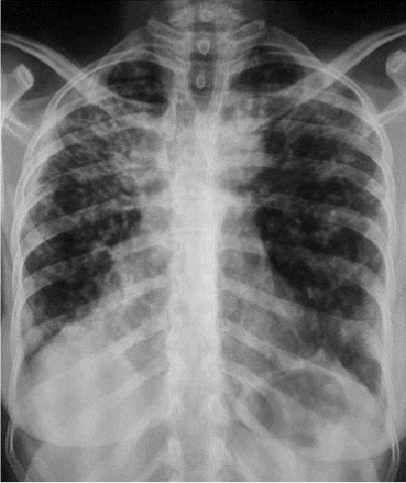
Chest radiograph of a patient with bronchiectasis diffus
